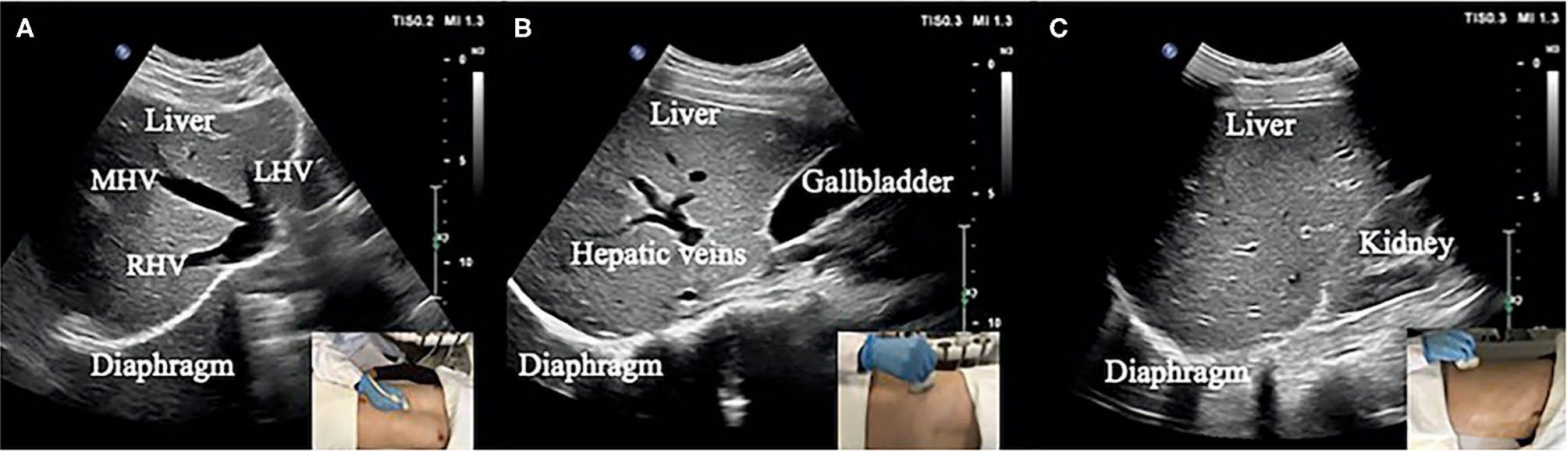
Three sections of ultrasound images. (A) Section I: Oblique section of the lower right costal arch through the second hepatic portal with the left hepatic vein (LHV), middle hepatic vein (MHV), and right hepatic vein (RHV) as anatomical markers. (B) Section II: Oblique section of the right intercostal passage through the first hepatic portal with the inferior vena cava, hepatic vein, and gallbladder as anatomical markers. (C) Section III: Sagittal section of the liver and right kidney with the right kidney and hepatorenal space as anatomical markers [16]
